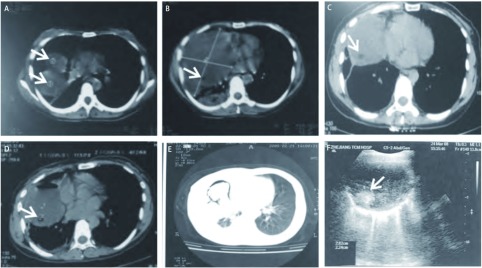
胸部CT及B超所见。A：（2月11日）右上叶肿块与纵膈成锐角，中间有肺组织分膈，肿块中间有高密度影（箭头所指）；B：（2月11日）右下块纵膈紧密相连，没有含气组织及脂肪间膈，且与纵膈呈钝角，肿块中间有低密度影（箭头所指）；C（3月5日）、D（3月24日）：右下肺肿块内始终存在一类圆形低密度影（箭头所指），CT值：- 61.2 Hu；E：液体抽取后，出现气体和固态物质夹杂的征象；F：B超检查示囊性病灶中可见一类园形高回声影，边界清楚（箭头所指） The appearance of chest CT and B ultrasound. A: (11th, February) there is a tumor which high density image inside and devided by lung tissue in right upper lobes (arrow); B: (11th, February) a tumor which low density image inside and without gas and fat tissue (arrow); C (5th, March), D (24th, March): a tumor which spherical low density image inside in right lower lobes (arrow), CT value: -61.2 Hu; E: After draw fluid, there appear gas and solid matter inclusion image; F: B ultrasound show there is a hyperechogenicity image in a cystica tumor (arrow)
